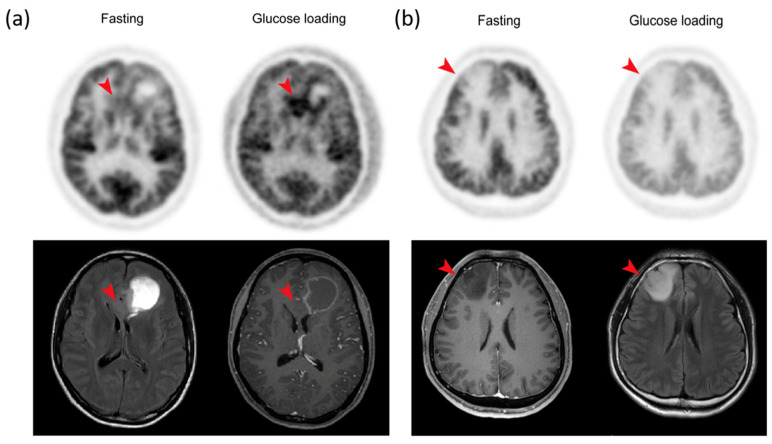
^18^F-FDG PET/CT (upper panel) and FLAIR and T1 enhanced MRI images (bottom panel) after fasting and glucose loading in a patient with (a) high-grade and (b) low-grade glioma. (a) Involvement of glioblastoma was pathologically confirmed in the left frontal lobe and corpus callosum (red arrow) in the glioblastoma. (b) Diffuse astrocytoma, IDH-mutant was pathologically confirmed in the right frontal lobe (red arrow).
